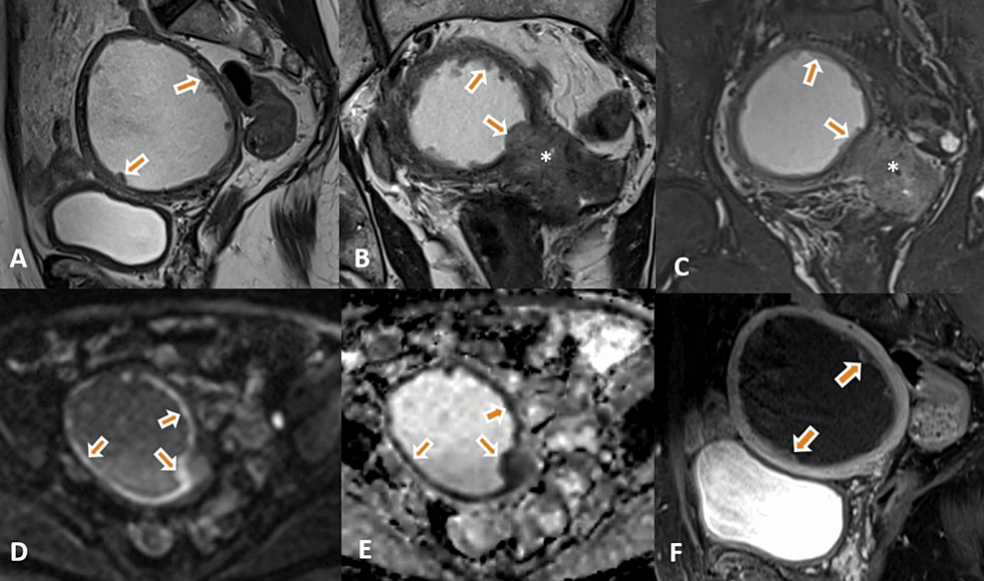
A-F: T2-weighted sagittal (A) and coronal (B) and STIR sagittal (C) images show irregular thickening and nodular deposits along the endometrial lining (thick arrows), in contiguity with the cervical lesion, and signal intensity to the primary cervical lesion (B, C asterisk). The thickened endometrium and nodular deposits show diffusion restriction (D, thick arrows) and corresponding low signal on the ADC image (E, thick arrows) with heterogenous enhancement on the axial post-contrast T1 FS image (F, thick arrows).
